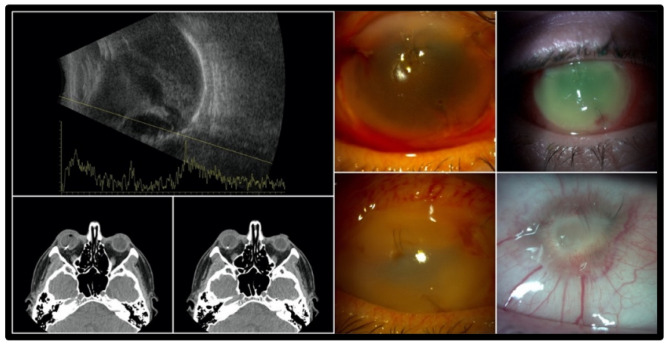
Imaging and clinical follow-up of the IOFB complicated with pan-ophthalmitis leading eventually to the eyeball phthisis (left side of the figure). Ultrasound examination (top left) showing dense vitreous hemorrhage and choroidal elevation. IOFB is visible as a hyperreflective signal in computer tomography (CT) (bottom left).
